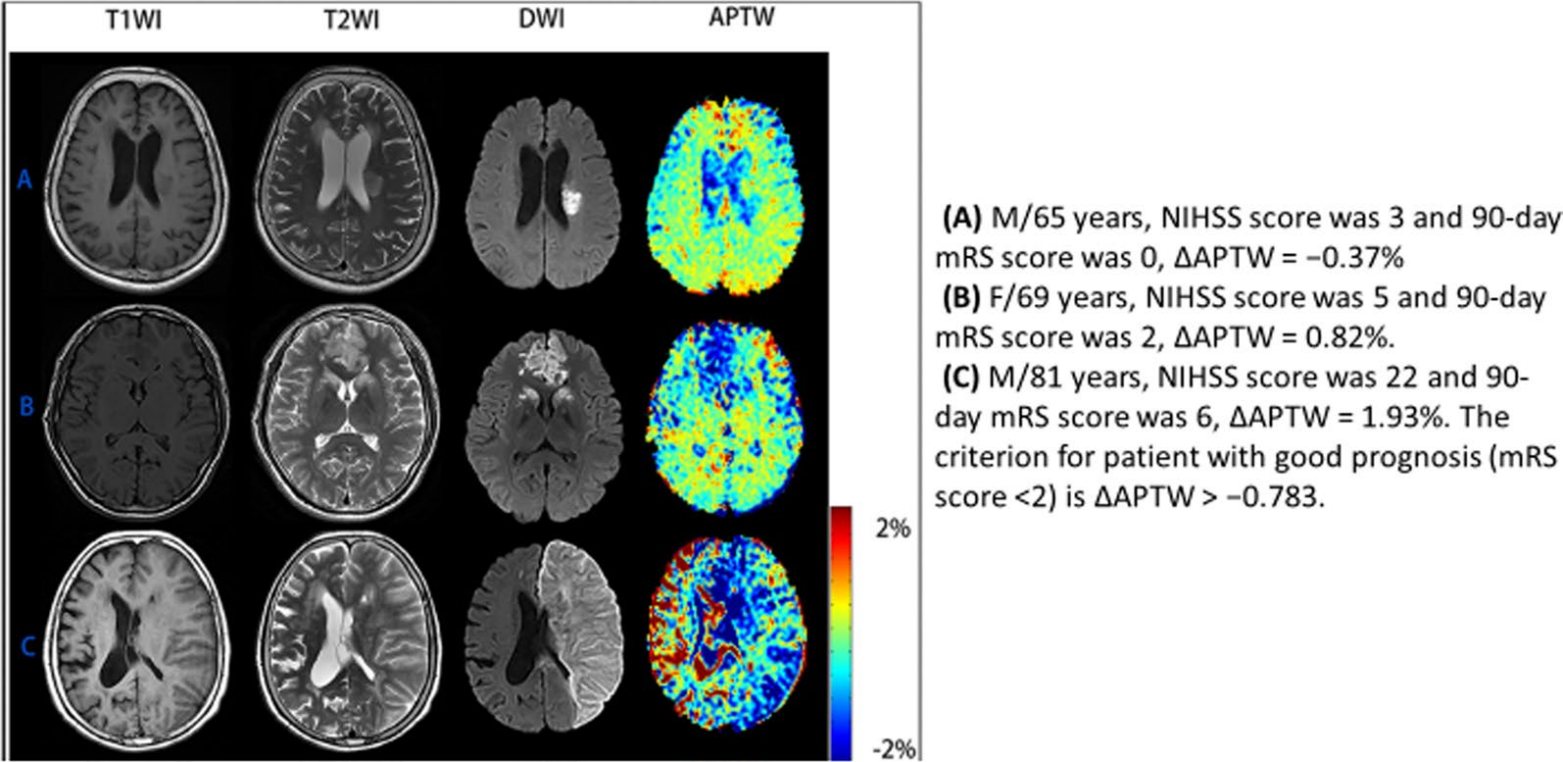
Conventional structural MR images (T1- and T2-weighted imaging [T1WI/T2WI]) in the first and second column, diffusion-weighted image (DWI) in the third column, and amide proton transfer weighted imaging (APTW) in the fourth column from the left. Each row corresponds to images from an individual patient with acute ischaemic infarct and differing clinical reports [93]. Figure reproduced according to the Creative Commons Attribution (CC BY) license from: Lin et al. [93]. Copyright © 2018 Lin, Zhuang, Shen, Xiao, Chen, Shen, Zong and Wu
